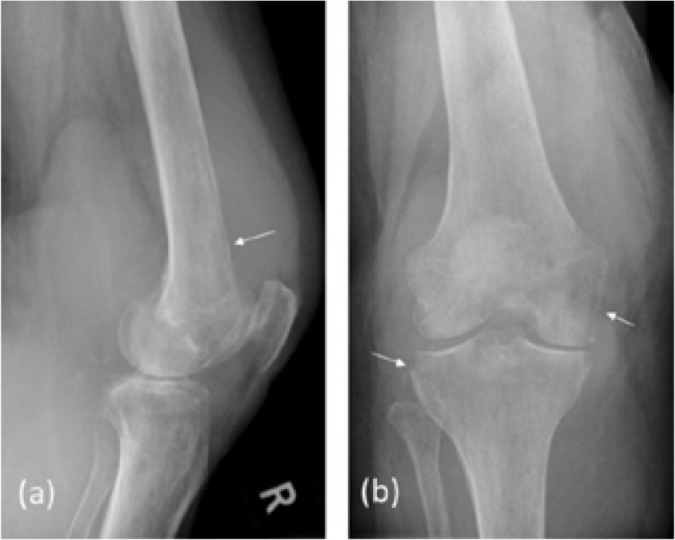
RIGHT Knee Lateral (a) and Frontal (b) X-ray. Gross supra and infrapatellar soft tissue swelling which had significantly increased in size. Loss of cortical definition to the anterior cortex of the distal femur (arrow in a) and medial femoral condyle suggestive of underlying erosions (arrows in b).
